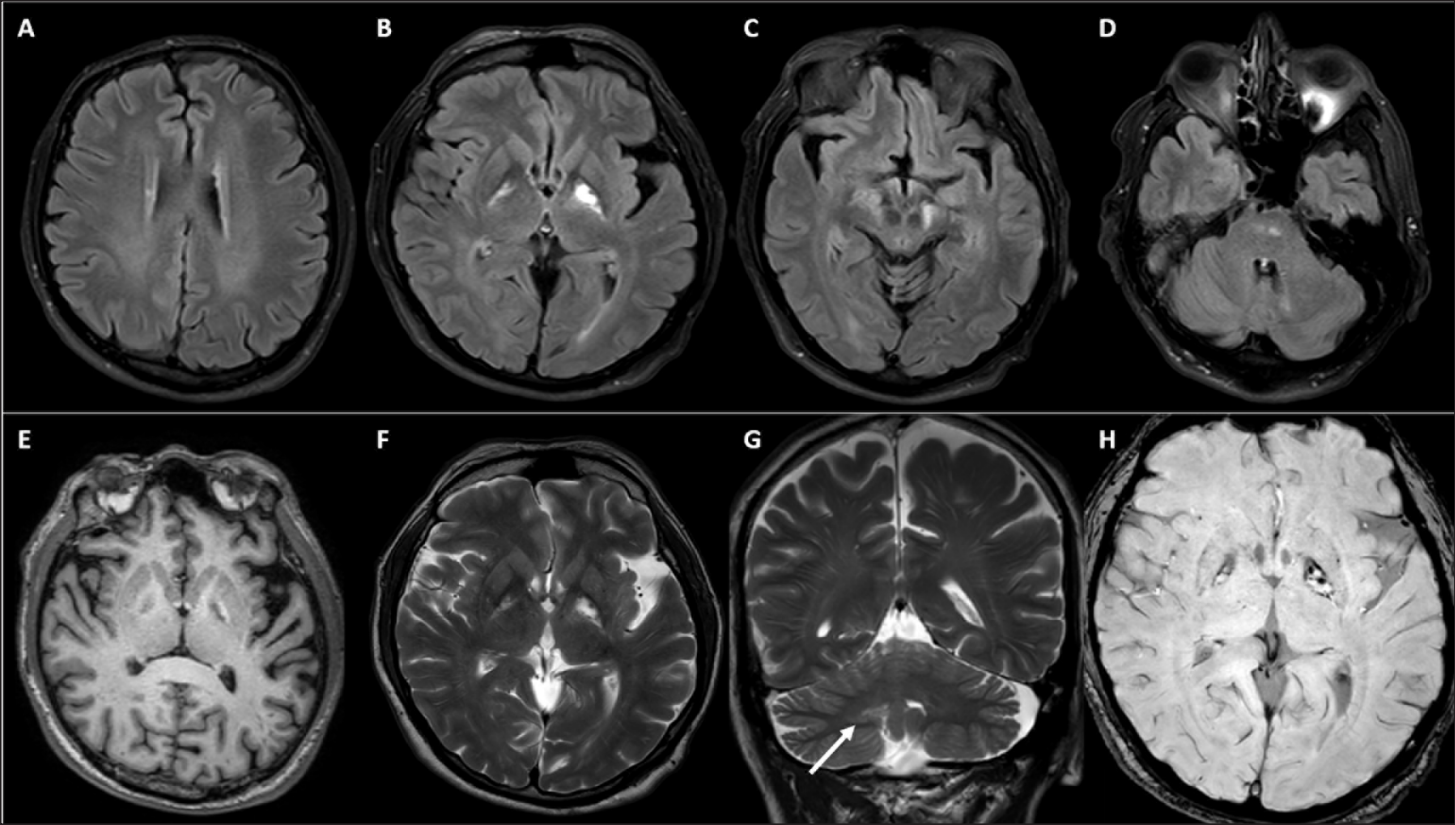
MRI of Patient-3. FLAIR axial images (A-D) showing hyperintensities along bilateral periventricular white matter and corticospinal tracts along internal capsules, midbrain and pons. Bilateral Globus pallidi appear hypointense on T1 (E) and hyperintense on T2 (F). Bilateral dentate nuclei appear slightly hyperintense on coronal T2 images (G, white arrow). Susceptibility weighted imaging show blooming in left more than right globus pallidi lesions (H).
